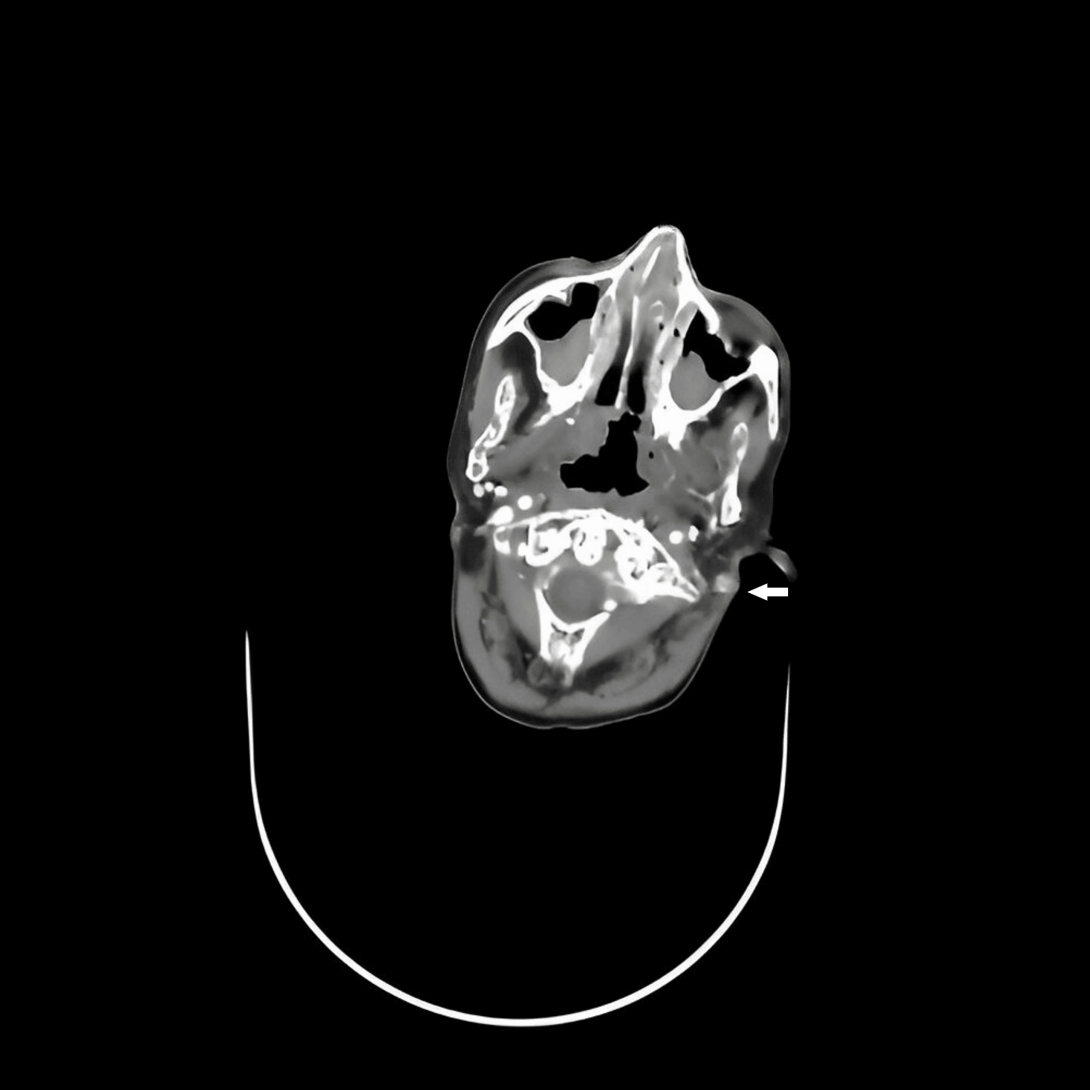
Medical imaging: cervico-facial CT scan revealing the lack of opacification of the left jugular vein and left-sided lateralized cavum parietal rigidity
